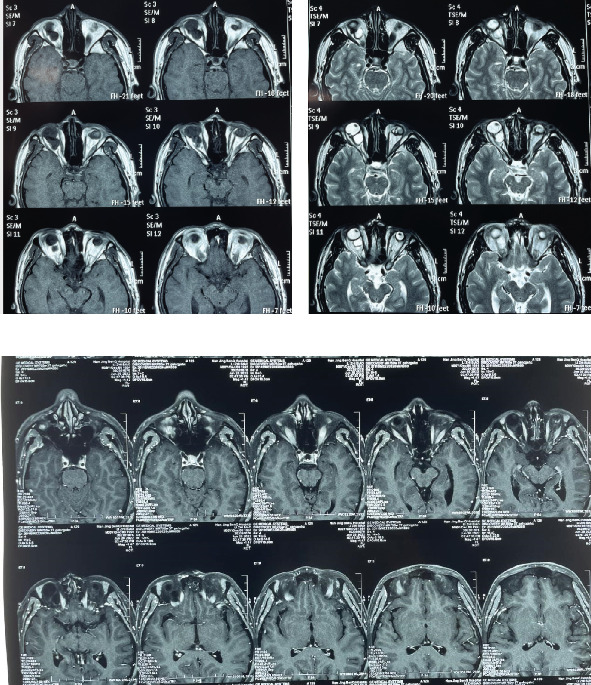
(a) Ordinary magnetic resonance imaging (MRI) of the orbit showing an irregular, well-defined mass measuring 13 × 9 mm, between the posterior inferior rectus muscle and the optic nerve in right eye. The mass compressed the optic nerve and displaced it to the nasal superior. The mass was isointense on T1-weighted images and hyperintense on T2 images. No communication between the bulbus oculi and the mass can be seen in the MRI findings. (b) Contrast-enhanced magnetic resonance imaging findings showing a fluid structure surrounded by a thin-walled cystic cavity.
